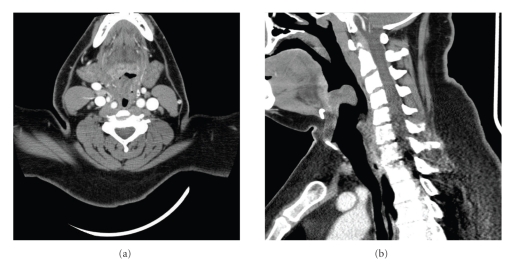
CT scan of the head and neck. (a) Axial and (b) sagittal CT scan images of the neck showing an epiglottic hematoma with moderate narrowing of the airway.
